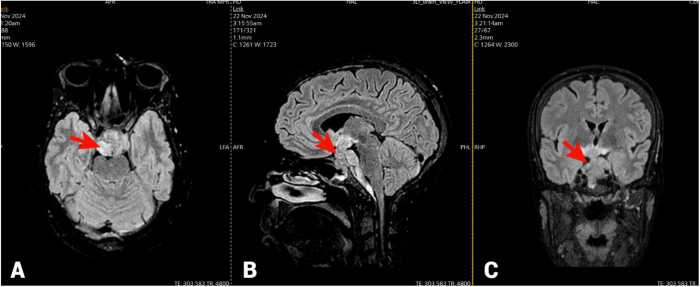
Brain MRI without IV contrast of the brain demonstrating: (A) axial, (B) sagittal, and (C) coronal views. There is a heterogeneous sellar and suprasellar mass with high signal intensity on T2W/FLAIR sequences measuring about (3.3 × 2.4 × 4 cm) and posterior extension measures 6 mm in thickness (red arrows). MRI: magnetic resonance imaging; IV: intravenous.
